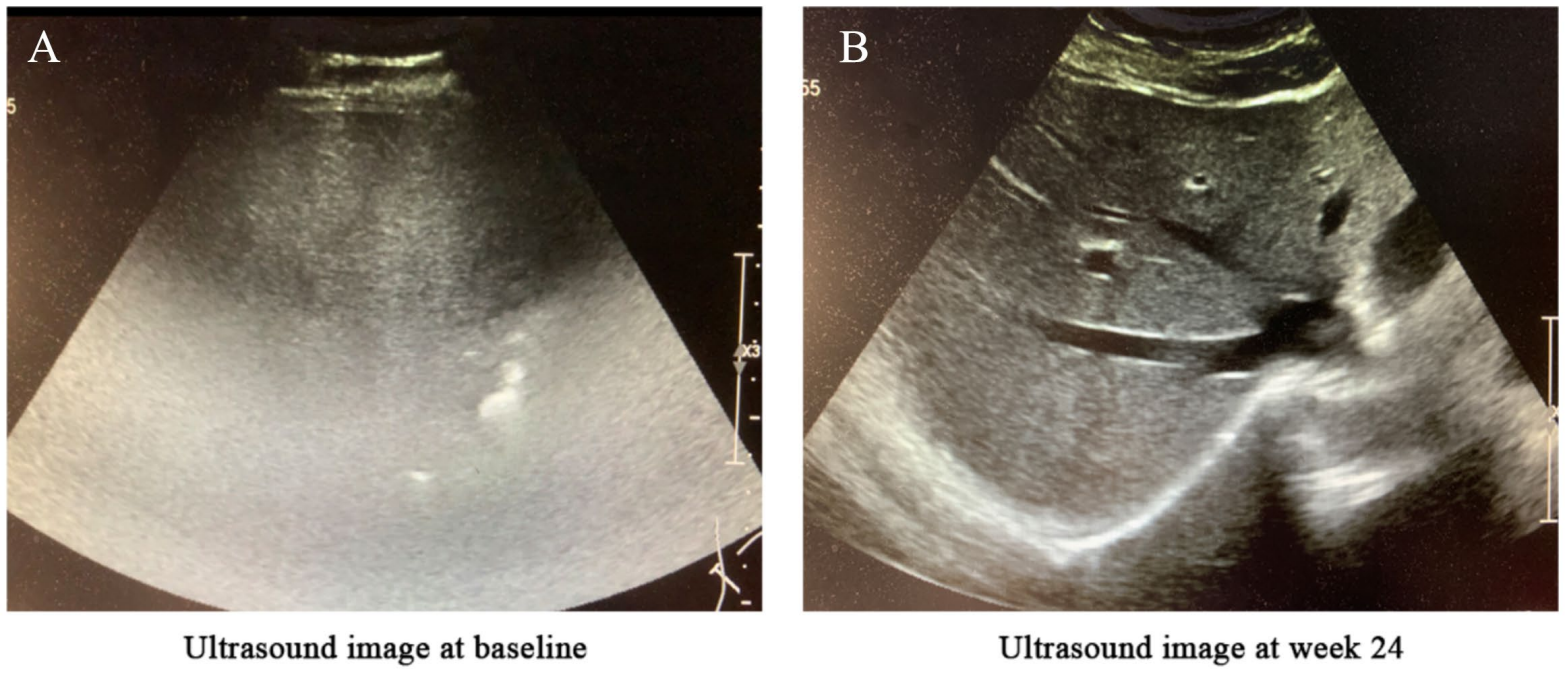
Changes in liver ultrasound imaging before and after treatment. (A) Baseline (before treatment): The hepatic parenchyma exhibited diffusely enhanced echogenicity, with unclear or absent visualization of intrahepatic blood vessels and significant attenuation of far-field echoes. The diaphragm was not visualized, suggesting severe fatty liver. (B) After 24 weeks of semaglutide treatment: The hepatic parenchyma showed diffusely increased echogenicity; however, the diaphragm and intrahepatic blood vessel walls were clearly visualized, indicating mild fatty liver.
